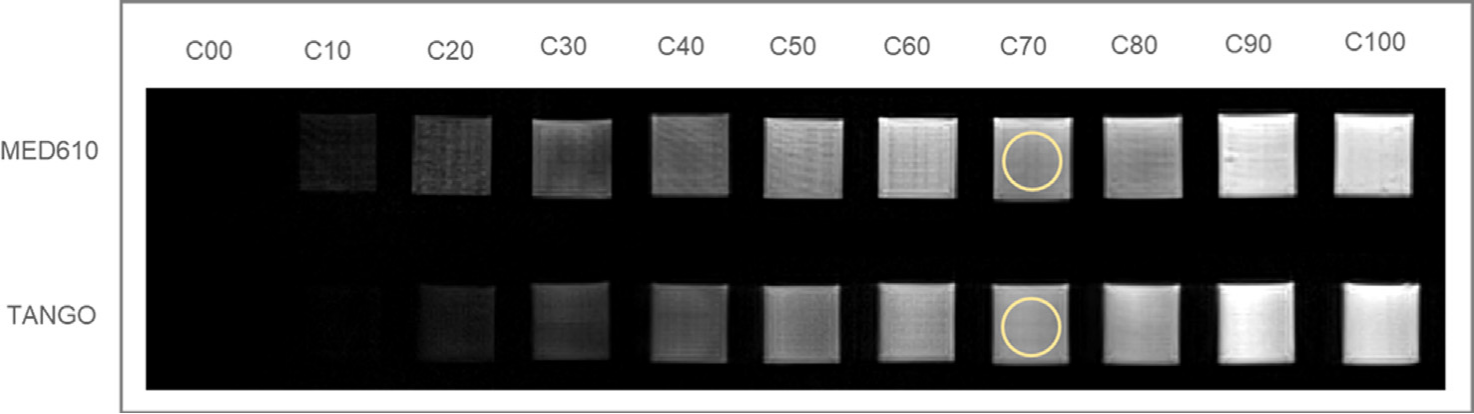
T1-w MR images of the samples. Two sets of 11 cubic samples were manufactured using two different materials for the main structure, MED610 (top) and TangoPlus (bottom). Each cubic object contains a different partial volume contribution of MRI-visible support material, ranging from C% = 0% (C00) to C% = 100% (C100). An example of the ROI size and positioning is shown in light yellow on sample C70. The structural artefacts visible in the MED610 samples are expected to arise from a problem with one of the two deposition nozzles used for MED610 in the printing head. This caused a partially imperfect deposition of the MED610 droplets in the printing process.
